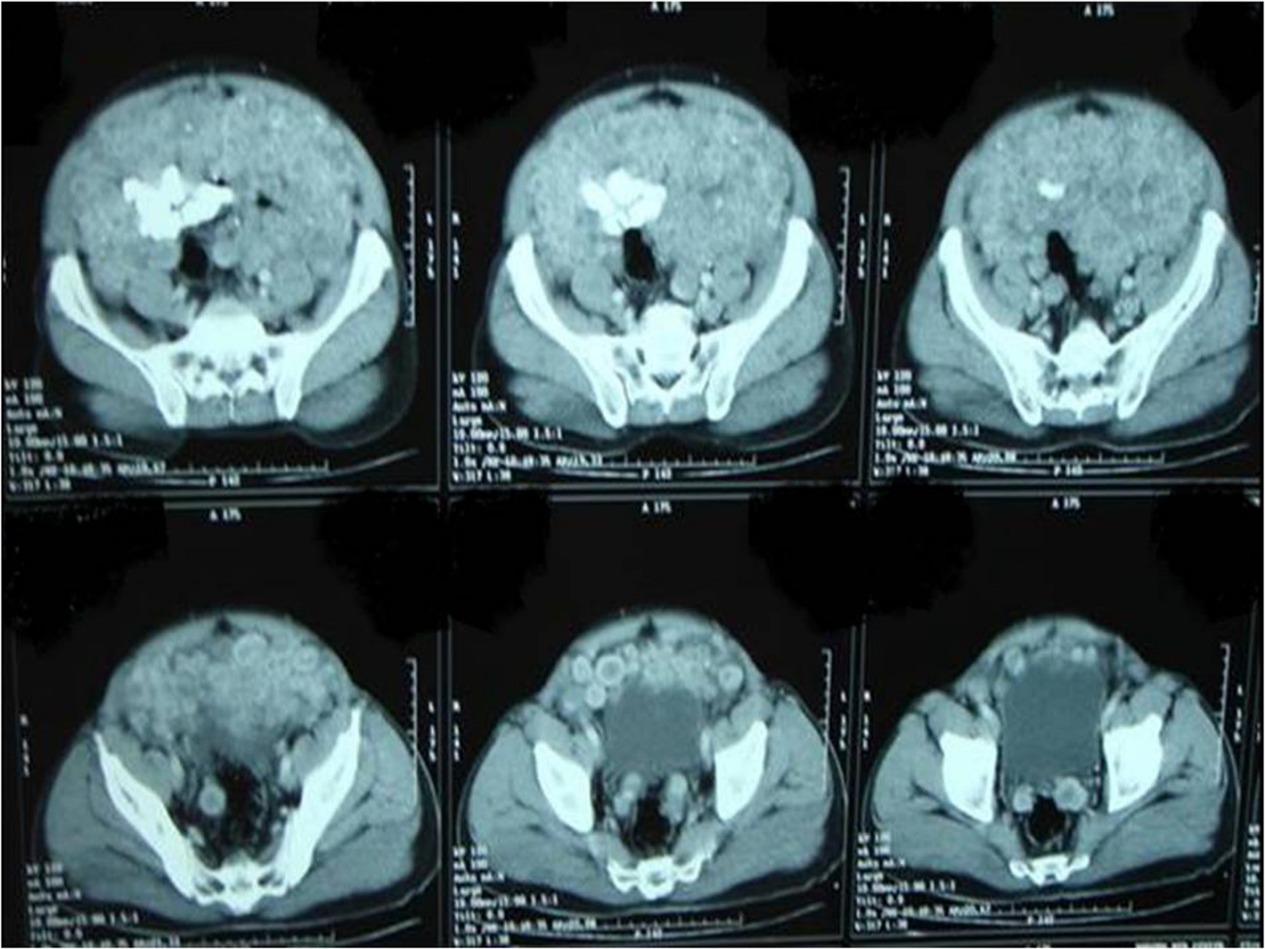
A computed tomography scan showed numerous nodules of different sizes (pelvis view).
